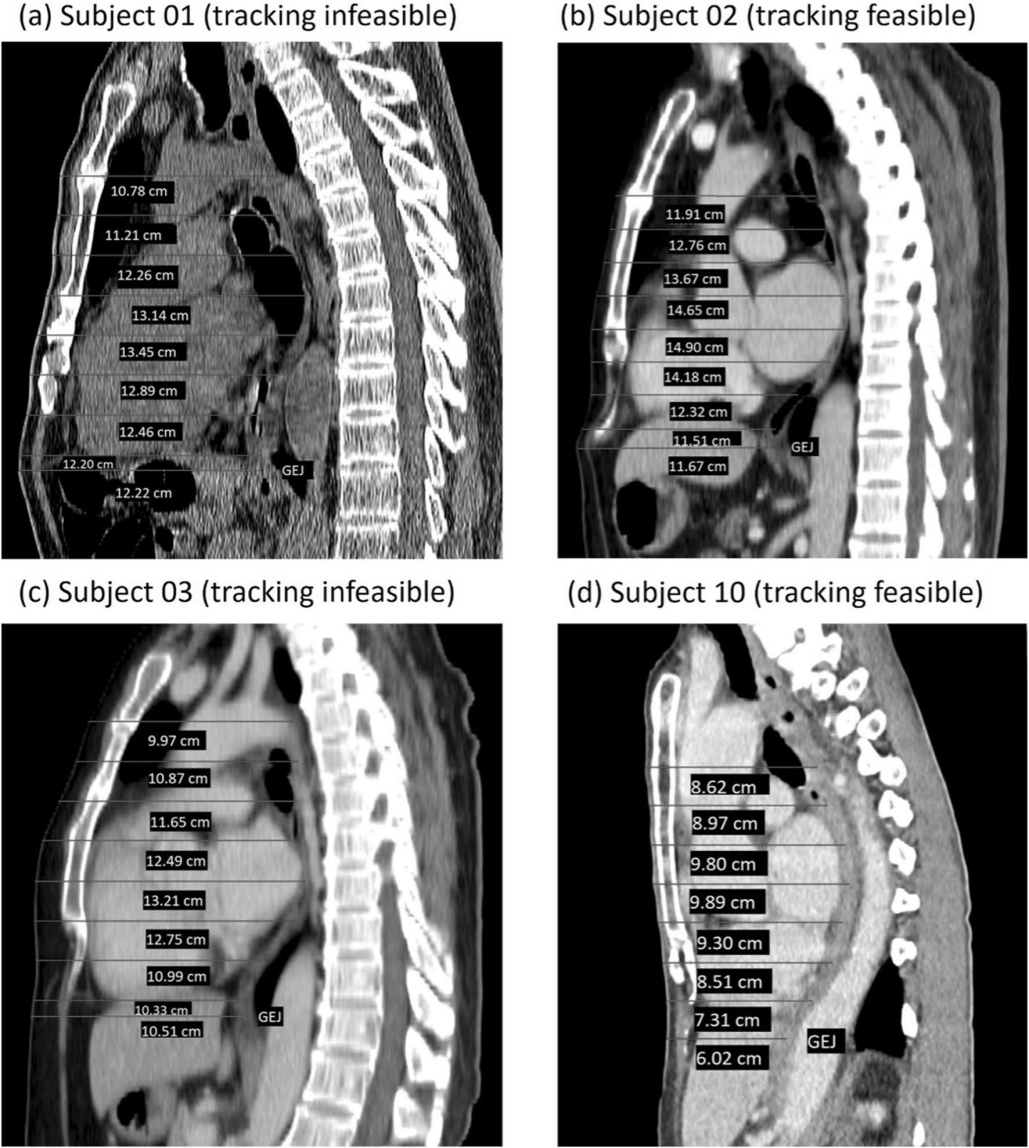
Distance between the esophagus and anterior chest wall in participants who had computed tomography before the trial. *GEJ* gastroesophageal junction.
